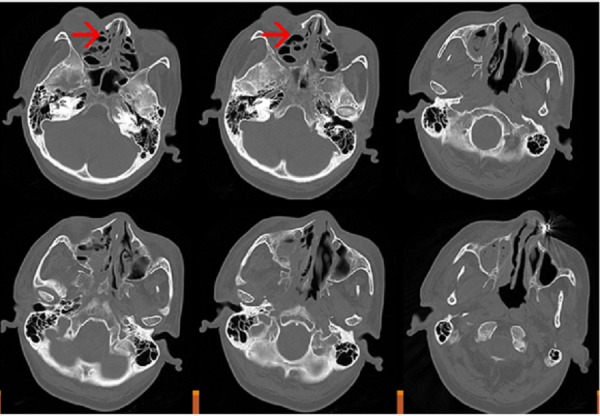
Contrast-enhanced computed tomography (CT) scan of paranasal sinuses is suggestive of soft tissue attenuation in right maxillary and frontal sinus.
